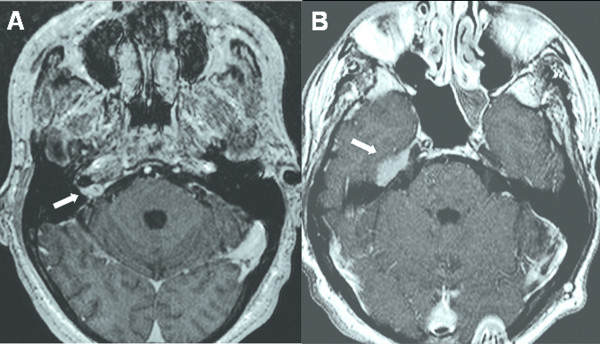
50-year-old man with biopsy-proven renal cell carcinoma to the right internal acoustic meatus (IAM). He was treated with 2500 cGy in 5 stages. (A) Axial MRI with contrast prior to radiosurgery showing the tumor at the IAM. *White arrow: tumor*. (B) Axial MRI with contrast 5 months after radiosurgery showing extension of disease cephalad. This area was treated with an additional 2400 cGy in 3 stages. *White arrow: tumor extension*.
